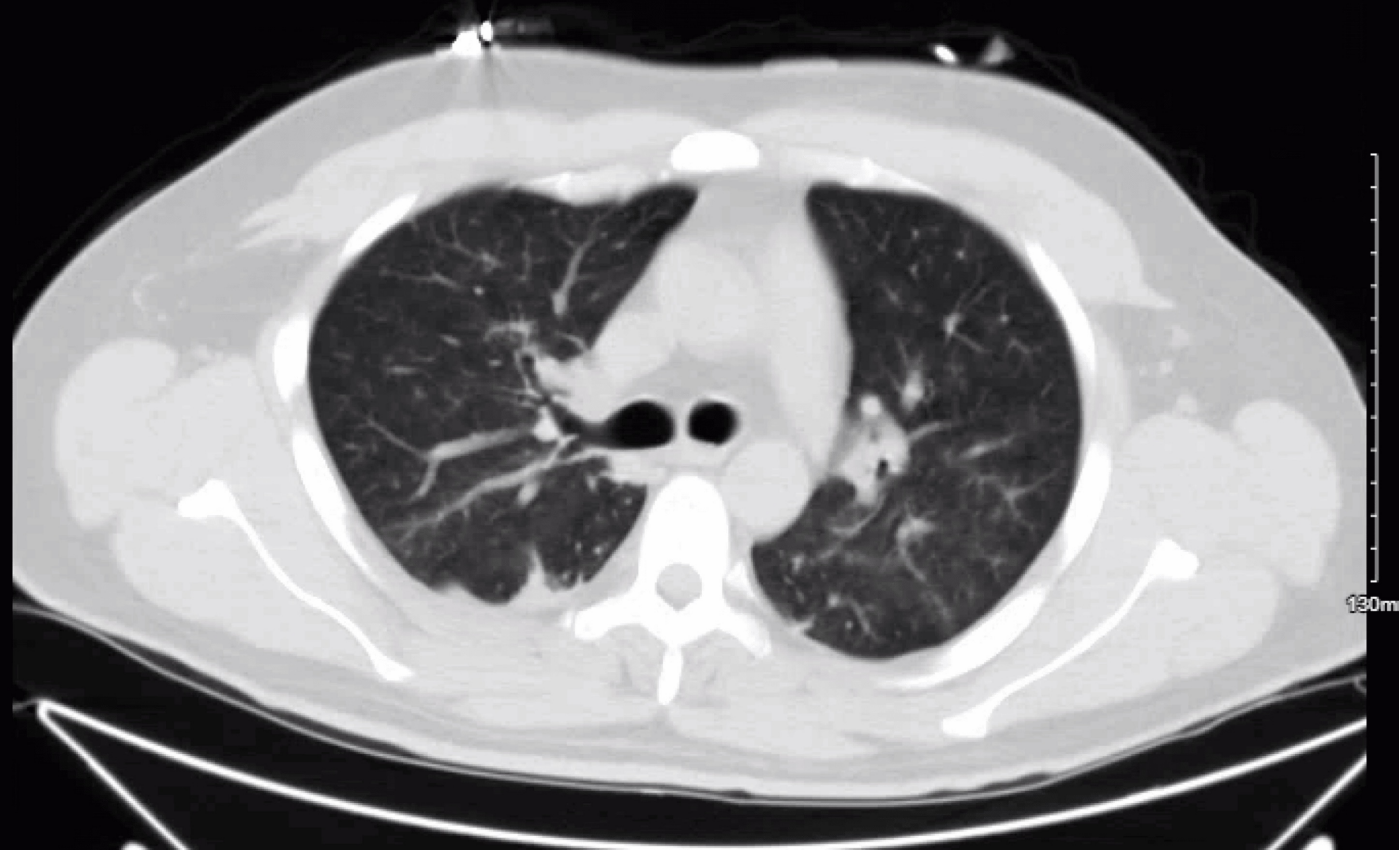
CT chest without contrast during the first hospitalization
